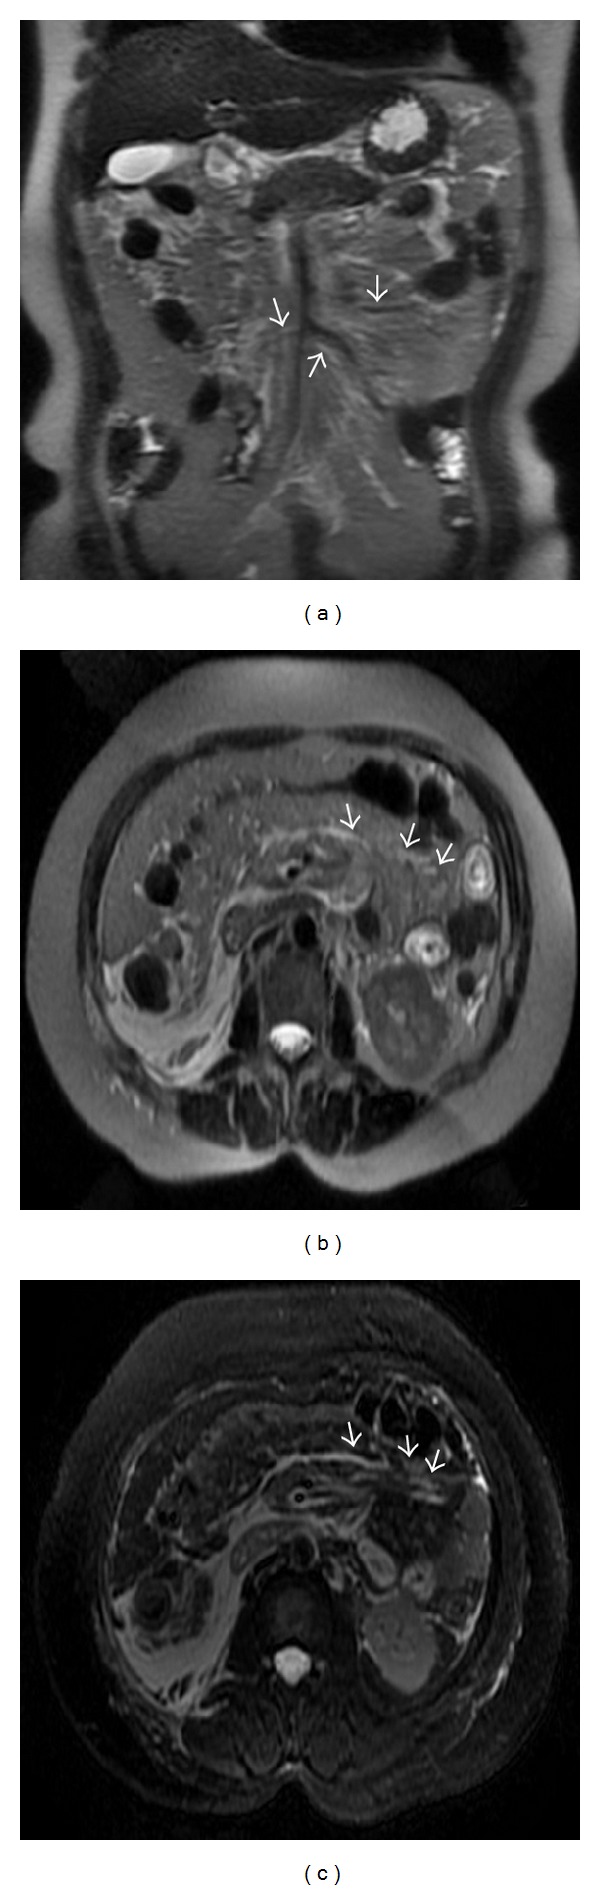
A 45-year-old male with moderate AP. The mesenterical edema and thickening on coronal SSFSE T2-weighted image (a), on axial SSFSE T2-weighted image (b), and on axial SSFSE T2-weighted with fat suppression image (c).
